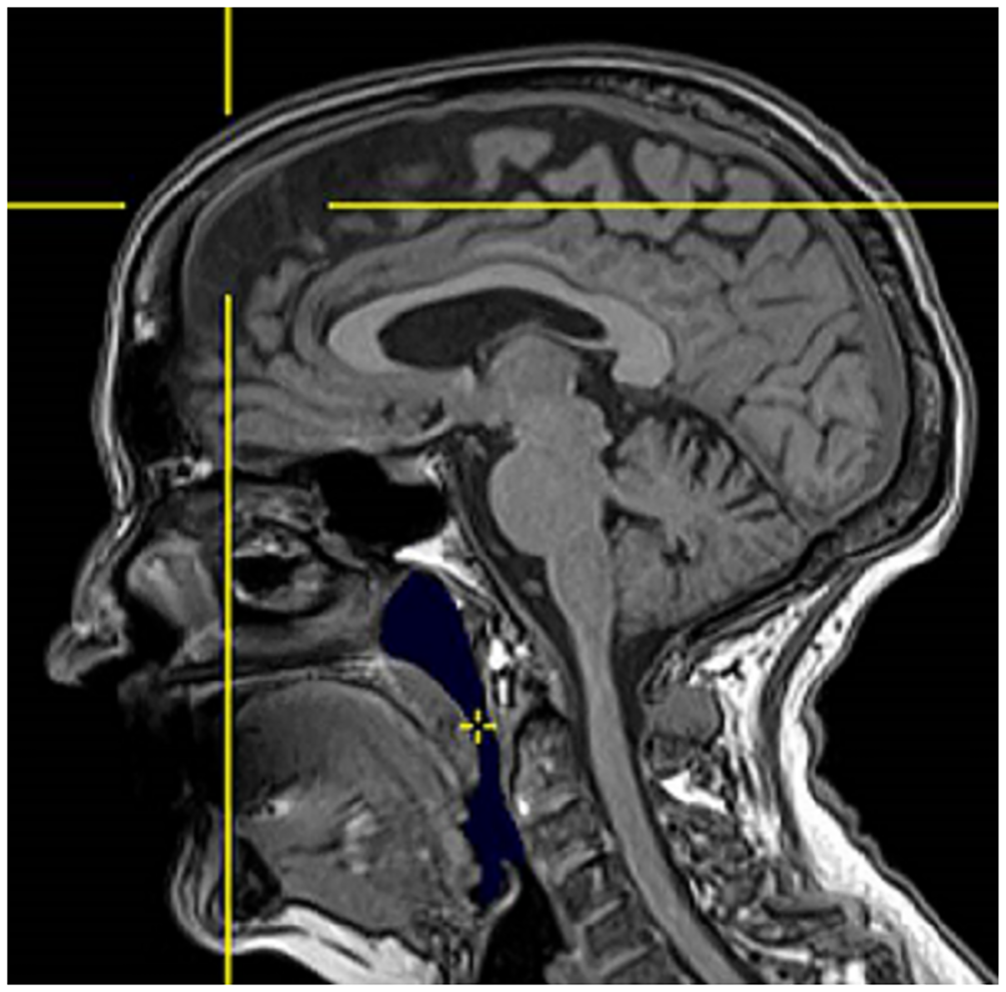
Sagittal slice of a three-dimensional T1-weighted magnetic resonance image acquisition in a single control subject. The semi-automatic segmented volume is shown in blue. The cross indicates the initial point of growth for the volume calculation.
